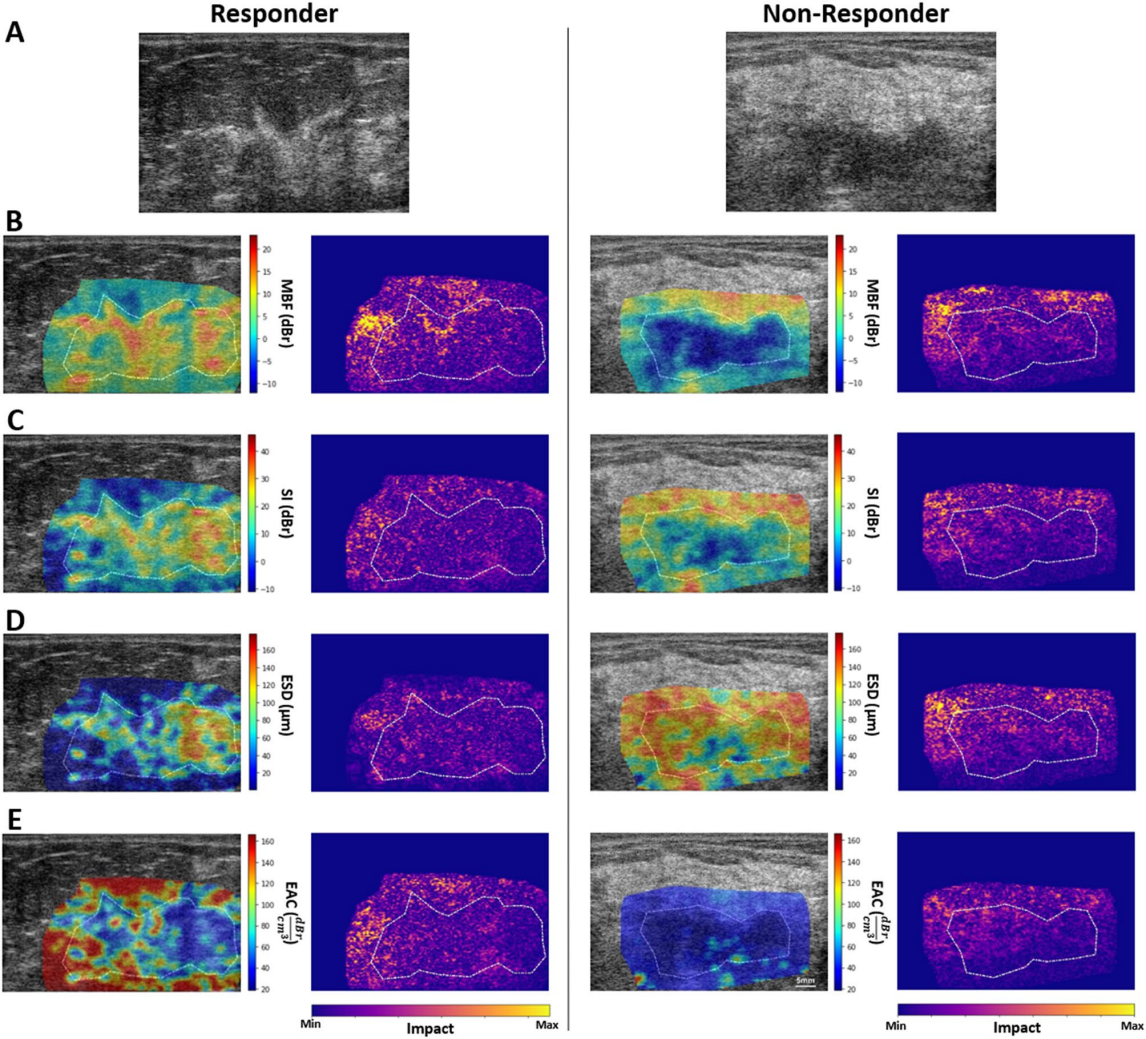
Ultrasound B-mode images (A), and parametric overlays of MBF (B), SI (C), ESD (D), and EAC (E) on B-mode images acquired at pre-treatment from a representative responder and non-responder to NAC, and the associated PDA maps visualizing the level of impact of different regions in each parametric image on the network’s decision (model 4 in Table 2). The tumor core has been outlined with white dashed line.
